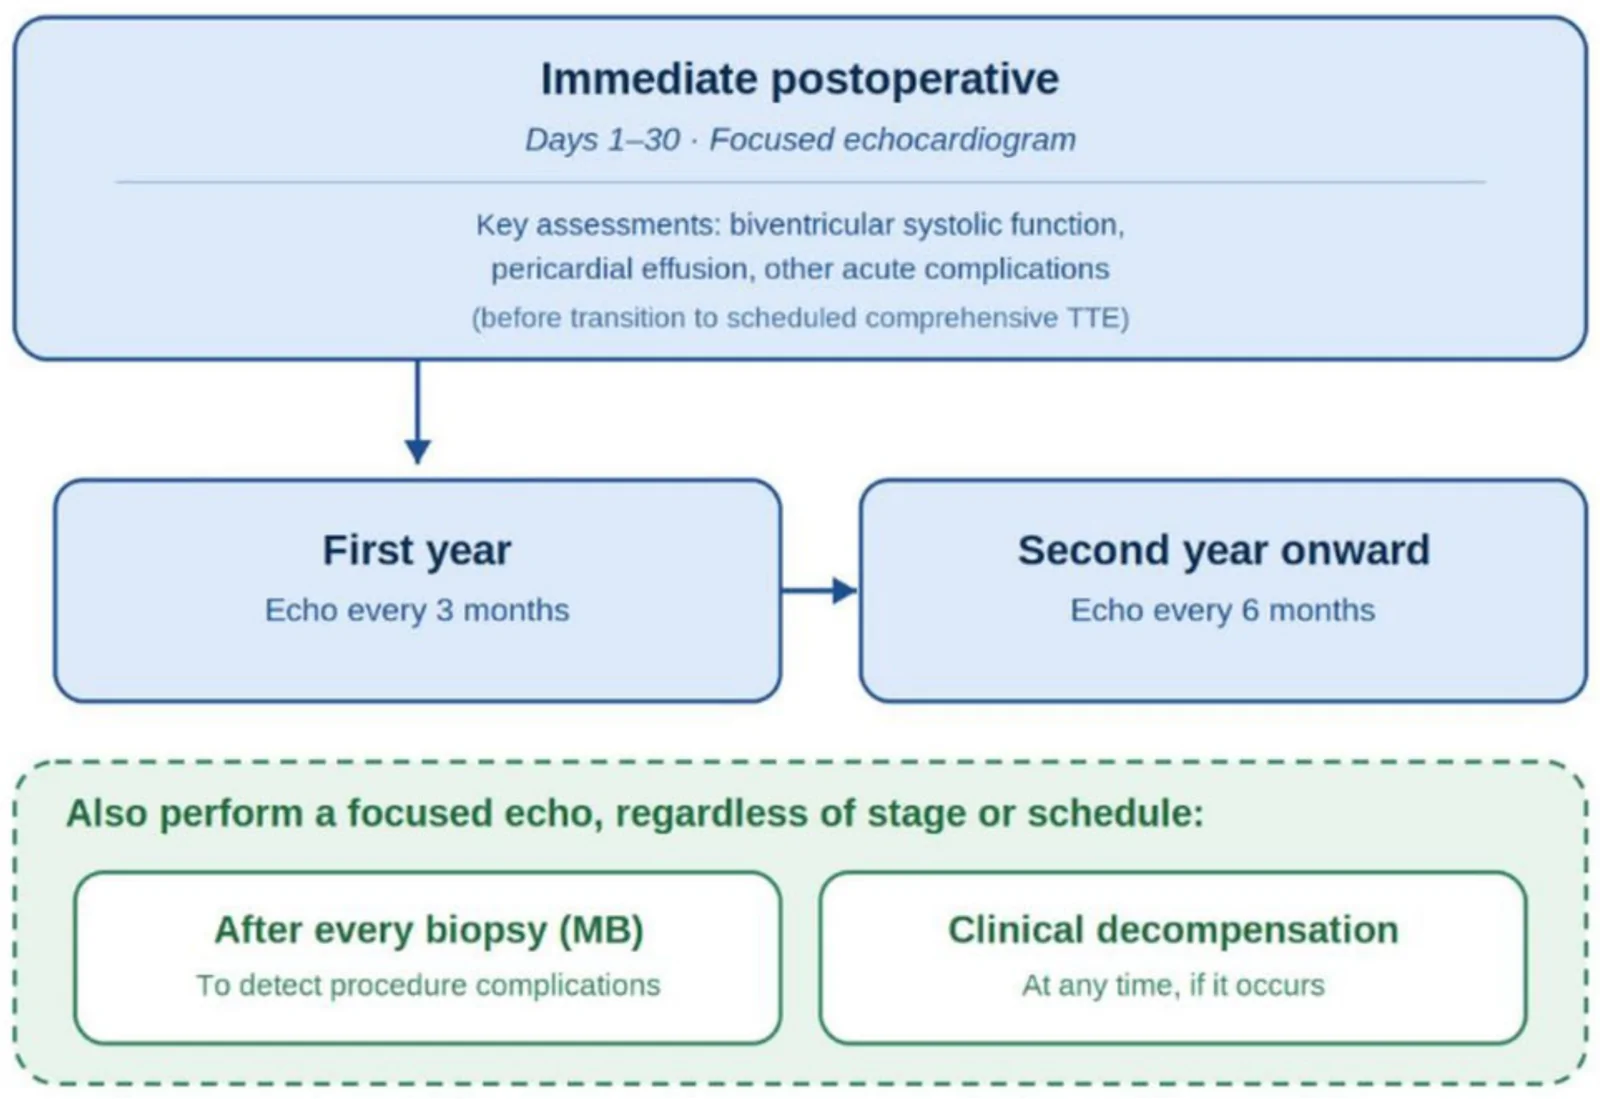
Recommended frequency of transthoracic echocardiography (TTE) during heart transplant follow-up. In addition to the scheduled intervals, a TTE should also be performed after every endomyocardial biopsy (MB) and in the presence of clinical decompensation. Abbreviations: TTE, transthoracic echocardiography; MB, myocardial biopsy.
